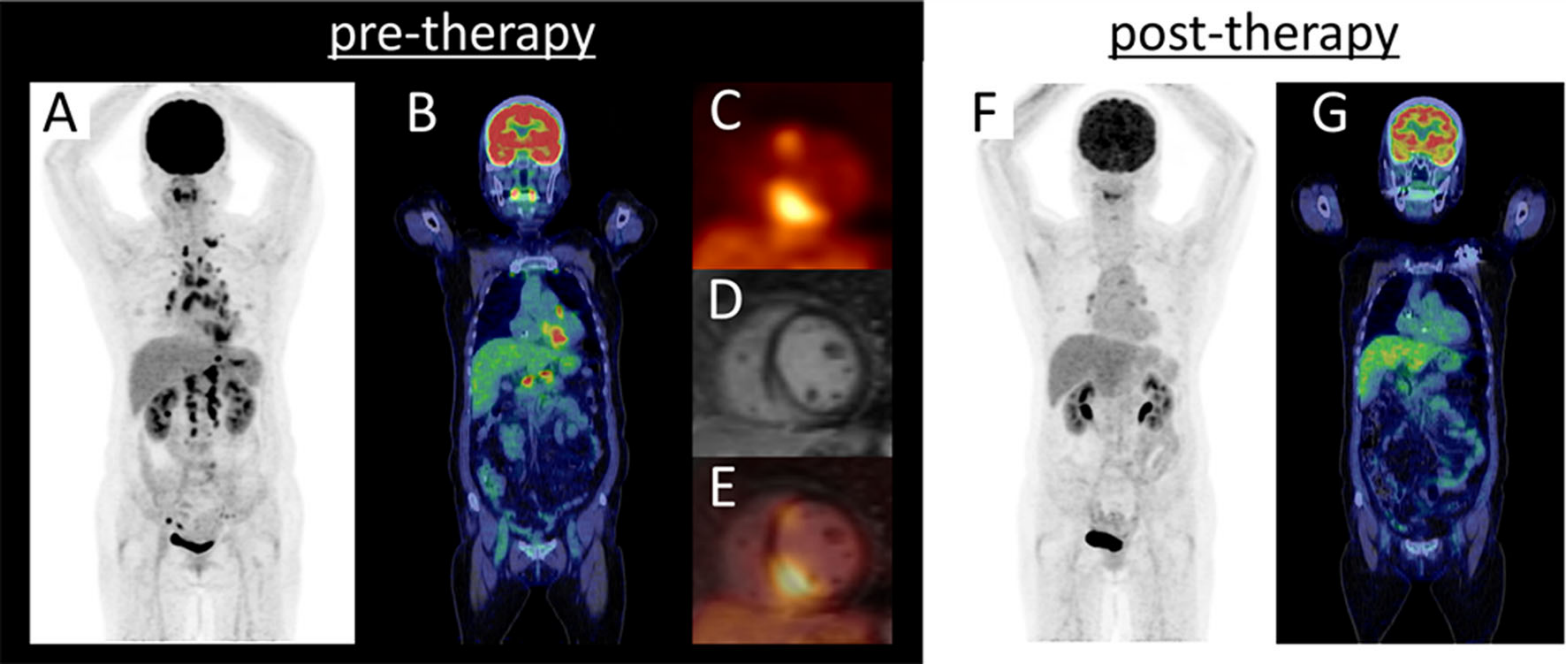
Representative case of cardiac sarcoidosis Maximum intensity projection (MIP) image of ^18^F-FDG PET (A), PET/CT coronal image (B), short-axis image of ^18^F-FDG PET (C), late gadolinium enhancement (LGE)-MRI (D), and fused image of ^18^F-FDG PET and LGE-MRI (E) at pre-therapy, MIP image of ^18^F-FDG PET (F) and PET/CT coronal image (G) at post-therapy (steroid 30 mg/1 month) are displayed. ^18^F-FDG PET detected focal cardiac uptake and multiple lymph node disease in the supraclavicular, mediastinum, hilum, abdominal, and pelvis region at pre-therapy. ^18^F-FDG uptake is seen at the same site of LGE-MRI abnormal intensity. At post-therapy, ^18^F-FDG uptakes were markedly lower. ^18^F-FDG is useful not only for diagnosis but also to confirm the effectiveness of treatments
